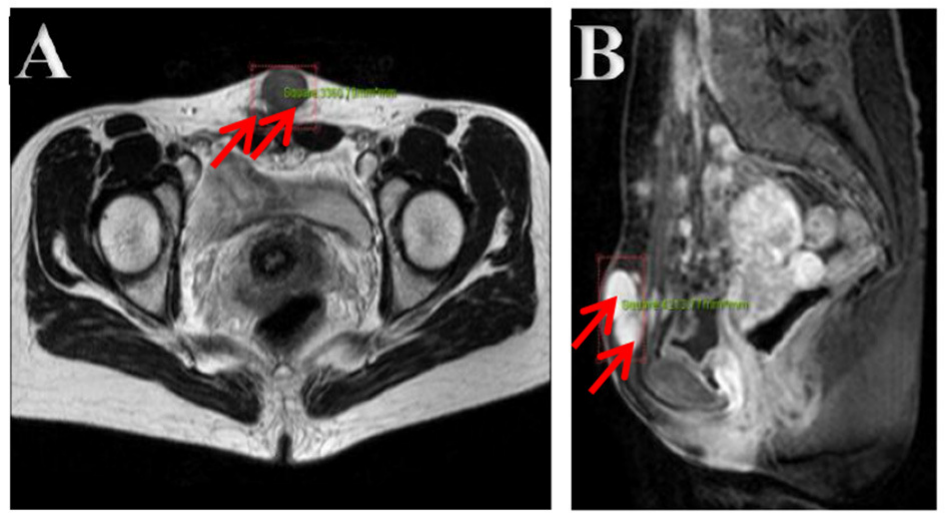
(A,B) Pelvic cavity magnetic resonance imaging. Multiple nodes in the abdominal wall, in the right iliac fossa, and behind the uterus, with lower T1- and T2-weighted image. The bigger showed homogeneous enhancement on enhancement images in the soft tissue of the lower abdominal wall.
